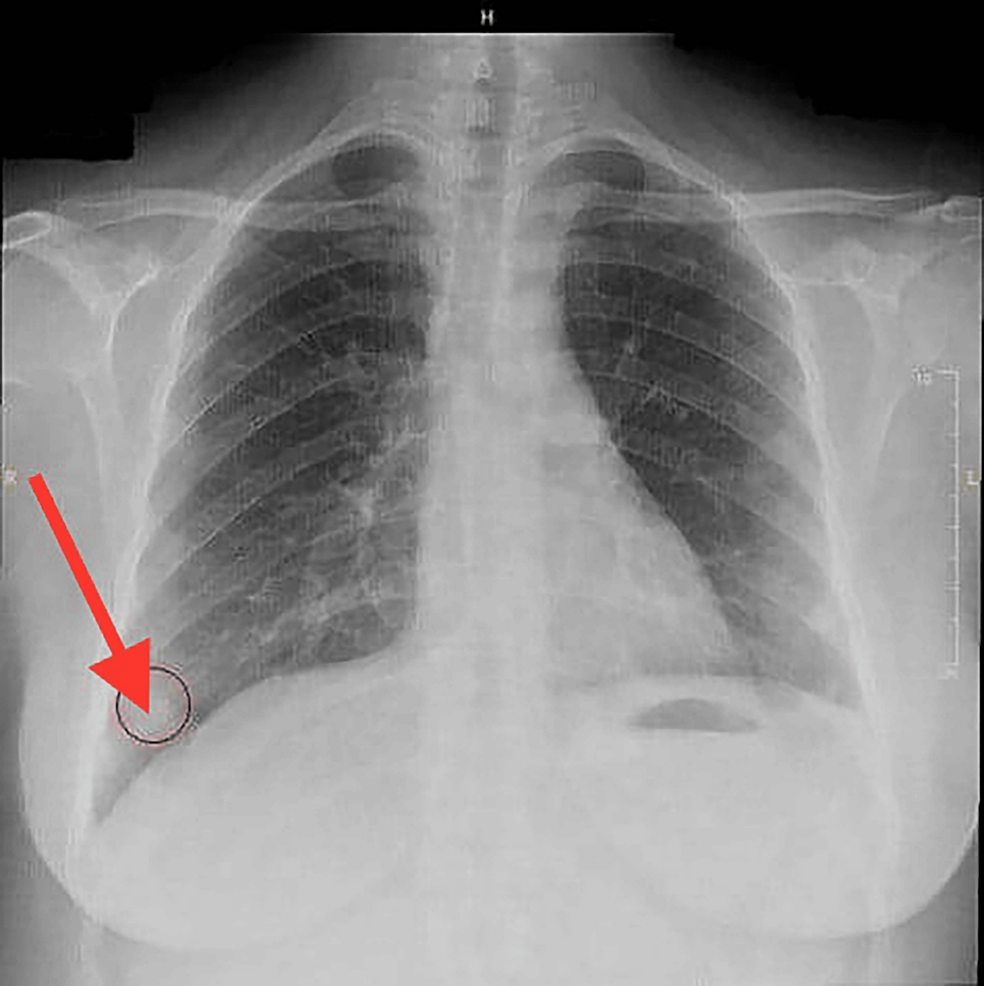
The chest X-ray of the patient showing a nodular opacity in the right lower lobe (July 22, 2023).
